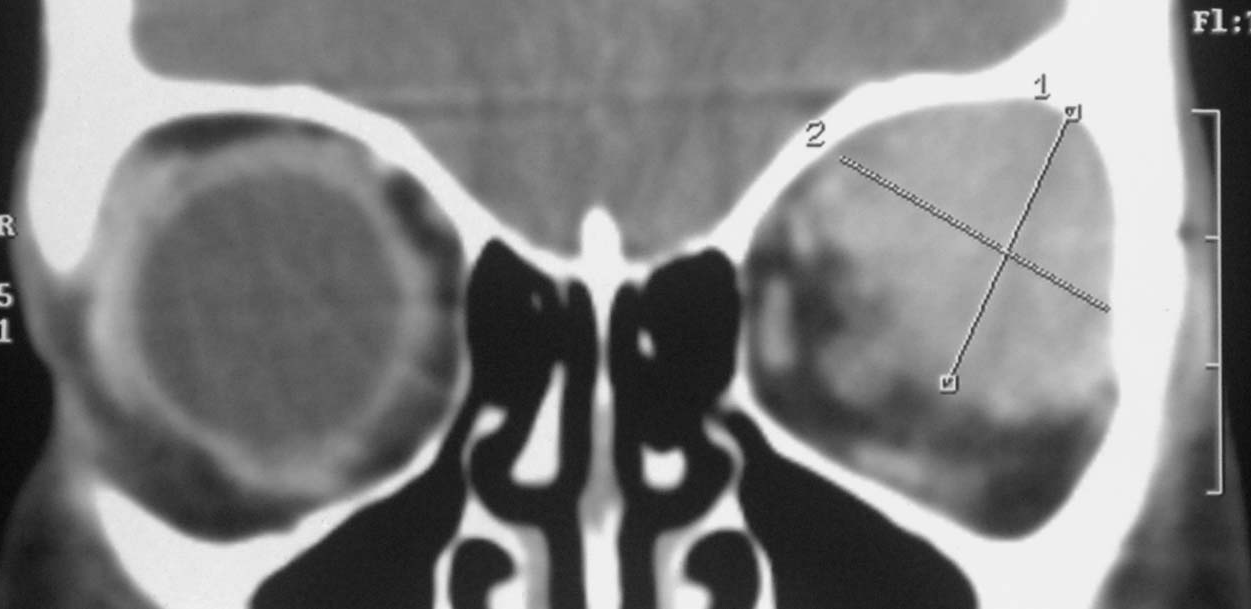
CT scan coronal view showed the superior and lateral rectus muscles could not be differentiated from the mass.
